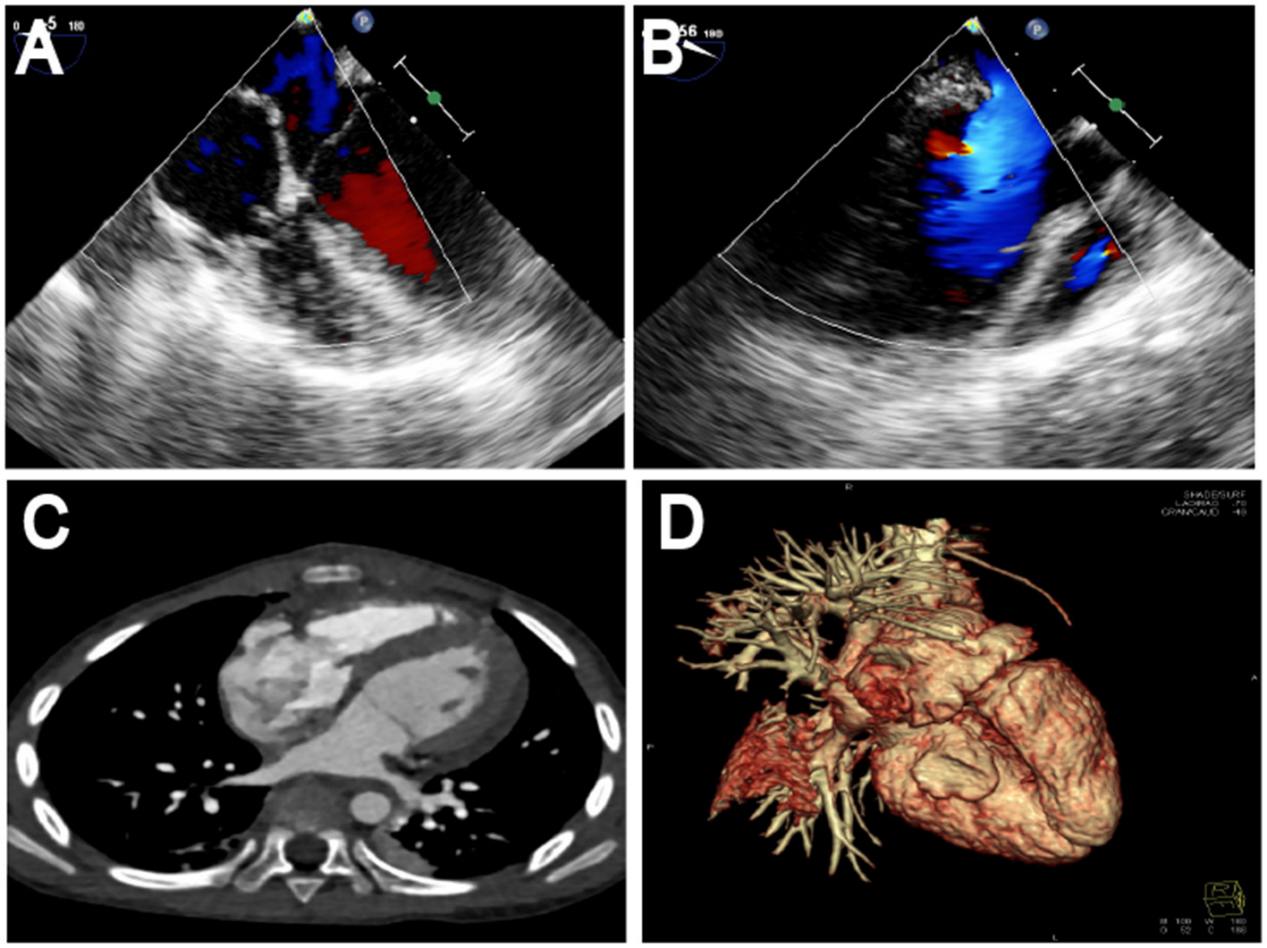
Follow-up. It showed no tricuspid regurgitation by intraoperative esophageal echocardiography showed no tricuspid regurgitation (A) and revealed no residual shunt for LVPW (B). The postoperative CT scan and reconstruction revealed successful surgery (C,D). LVPW, left ventricular posterior wall; CT, computed tomography.
